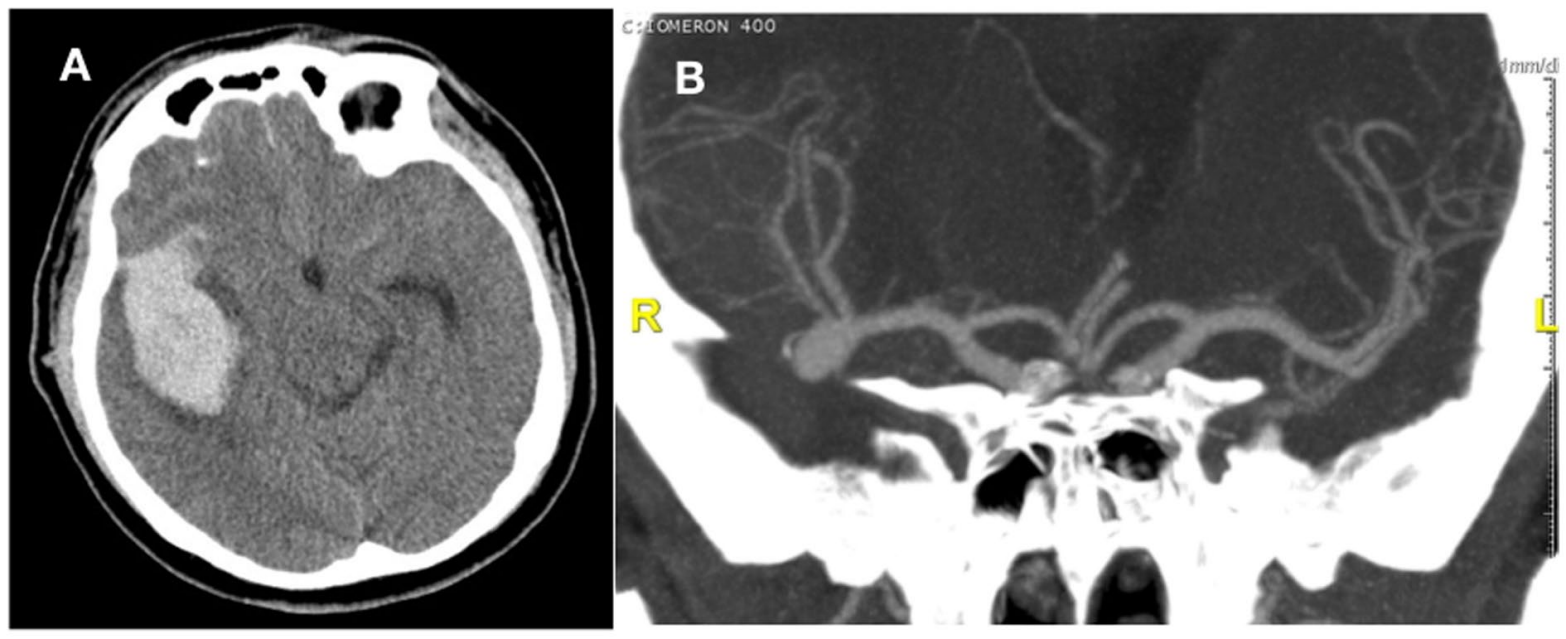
(A) CT scan with intracerebral hematoma. (B) CTA before surgery with an aneurysm on the trifurcation of the Middle cerebral artery (MCA).
